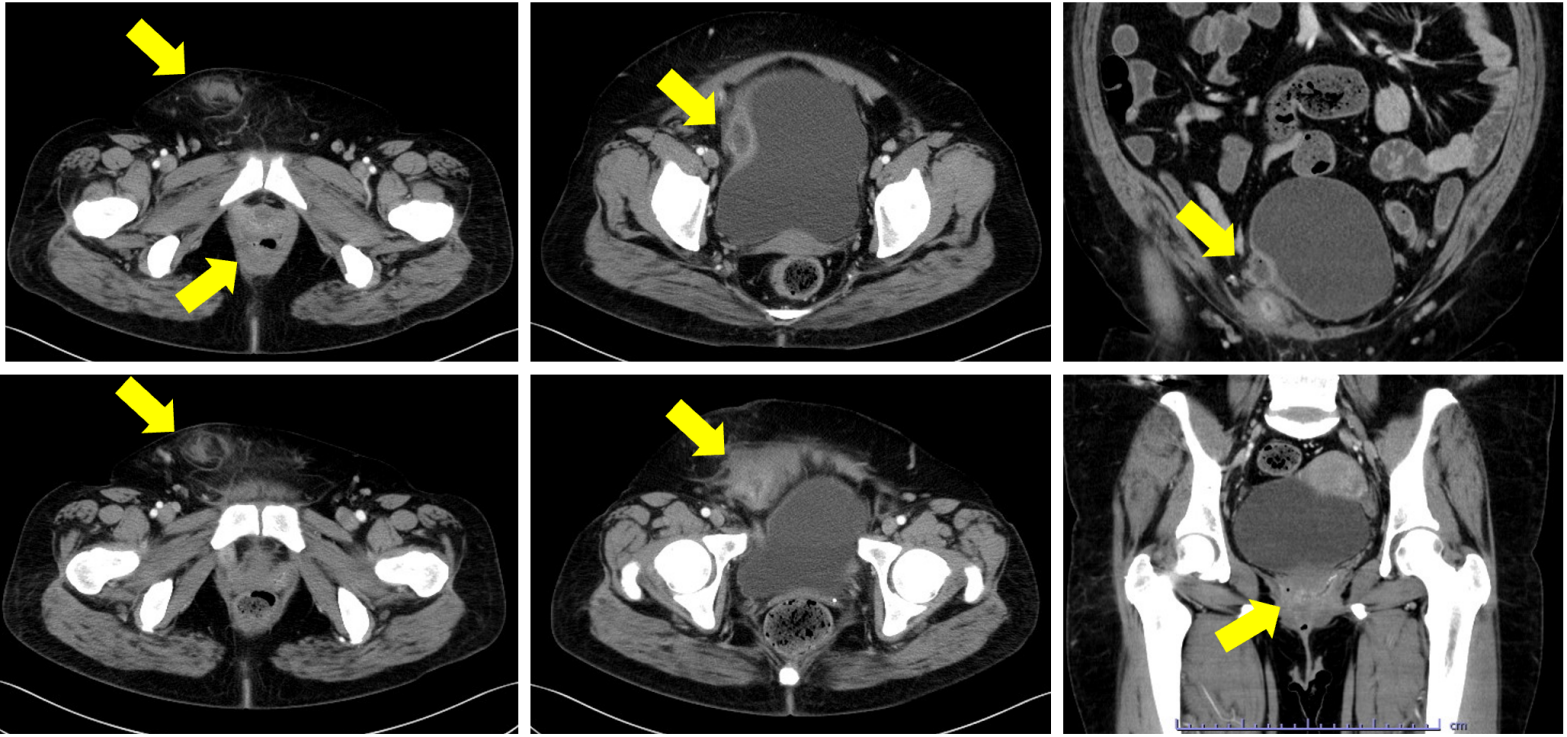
Contrast‐enhanced CT showing low‐density areas with ring enhancement suggestive of the abscess (arrow) in the right inguinal region, abdominal wall, lateral bladder cavity, and perivaginal area along the TVT insertion site. [Colour figure can be viewed at wileyonlinelibrary.com]
